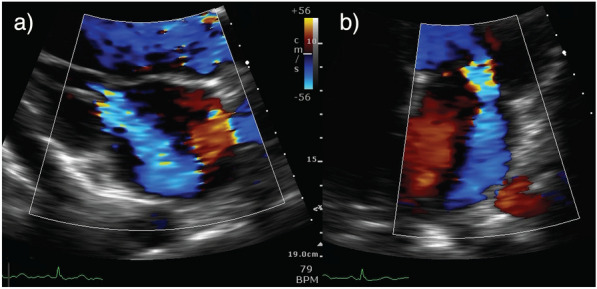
Case 3—Acute on chronic MR. a Posteriorly directed MR jet on parasternal long-axis (PLAX) view; b MR jet on apical 4 chamber (A4C) view.
